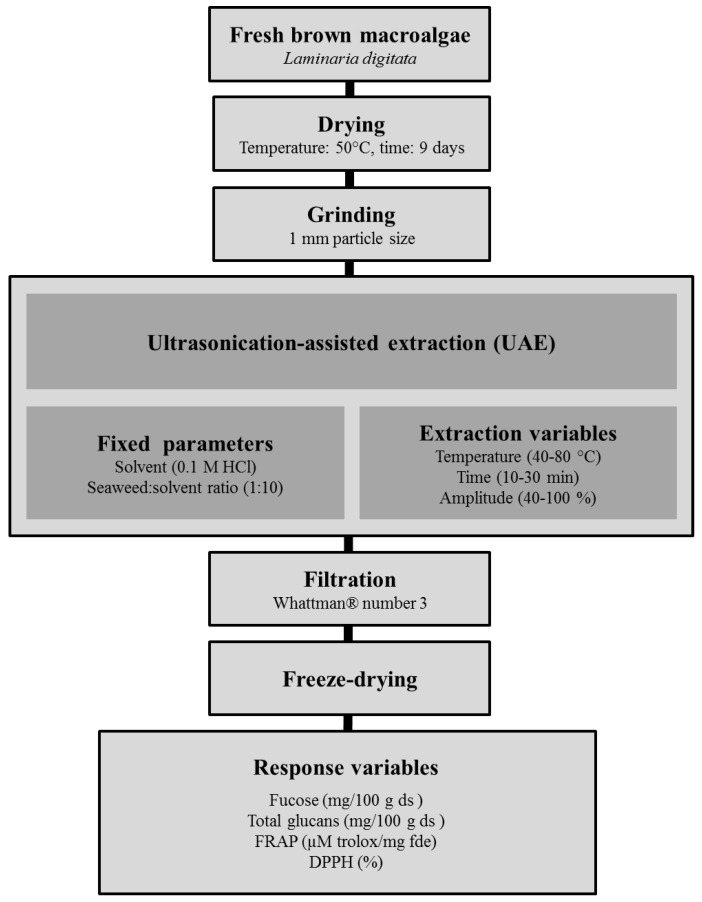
Scheme summarizing the pre-treatments of the fresh macroalgae and the ultrasound-assisted extraction (UAE) used to generate seaweed extracts.
